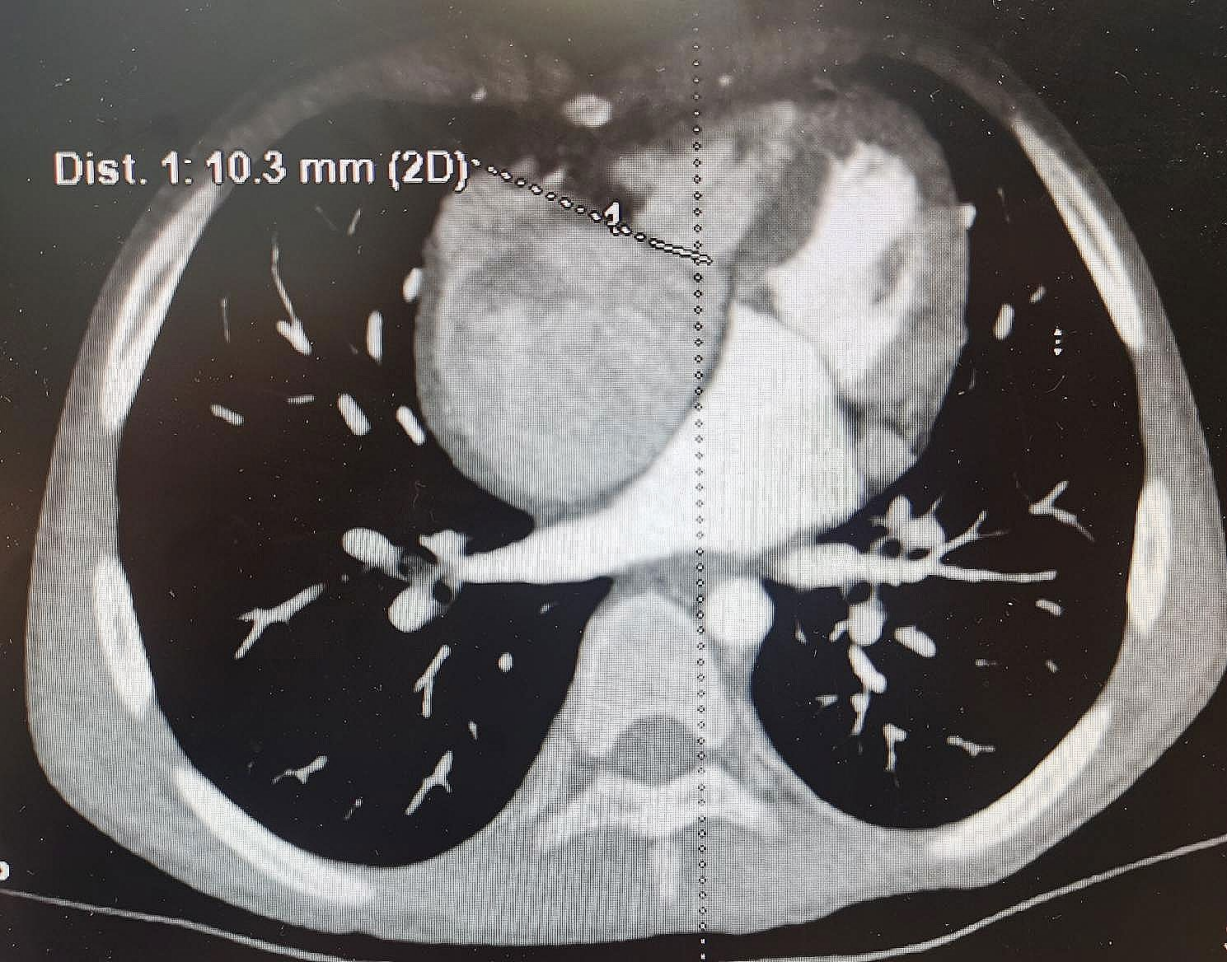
CT cardiac angiography showed that the right atrium was enlarged, the right ventricle was small, the diameter of Tricuspid annulus was 1.03 cm
